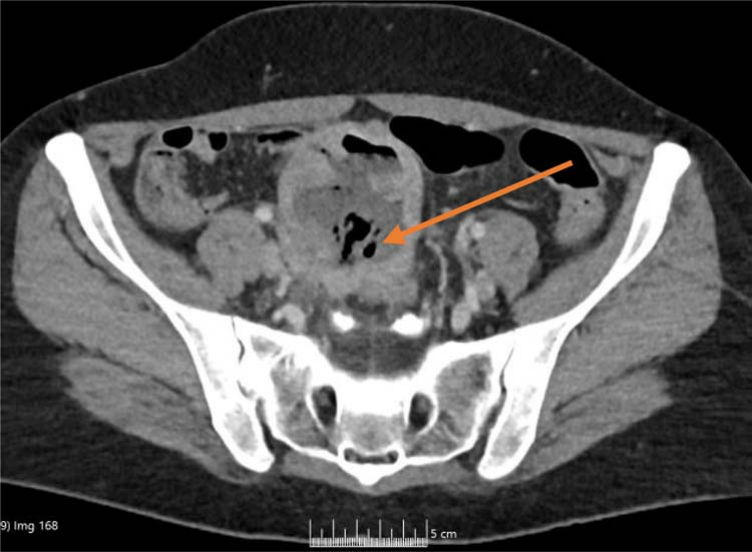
CT axial image, with arrow showing the midline lower abdominal/pelvic thick-walled fluid and gas-filled structure that measured 5 × 7 × 6 cm.
